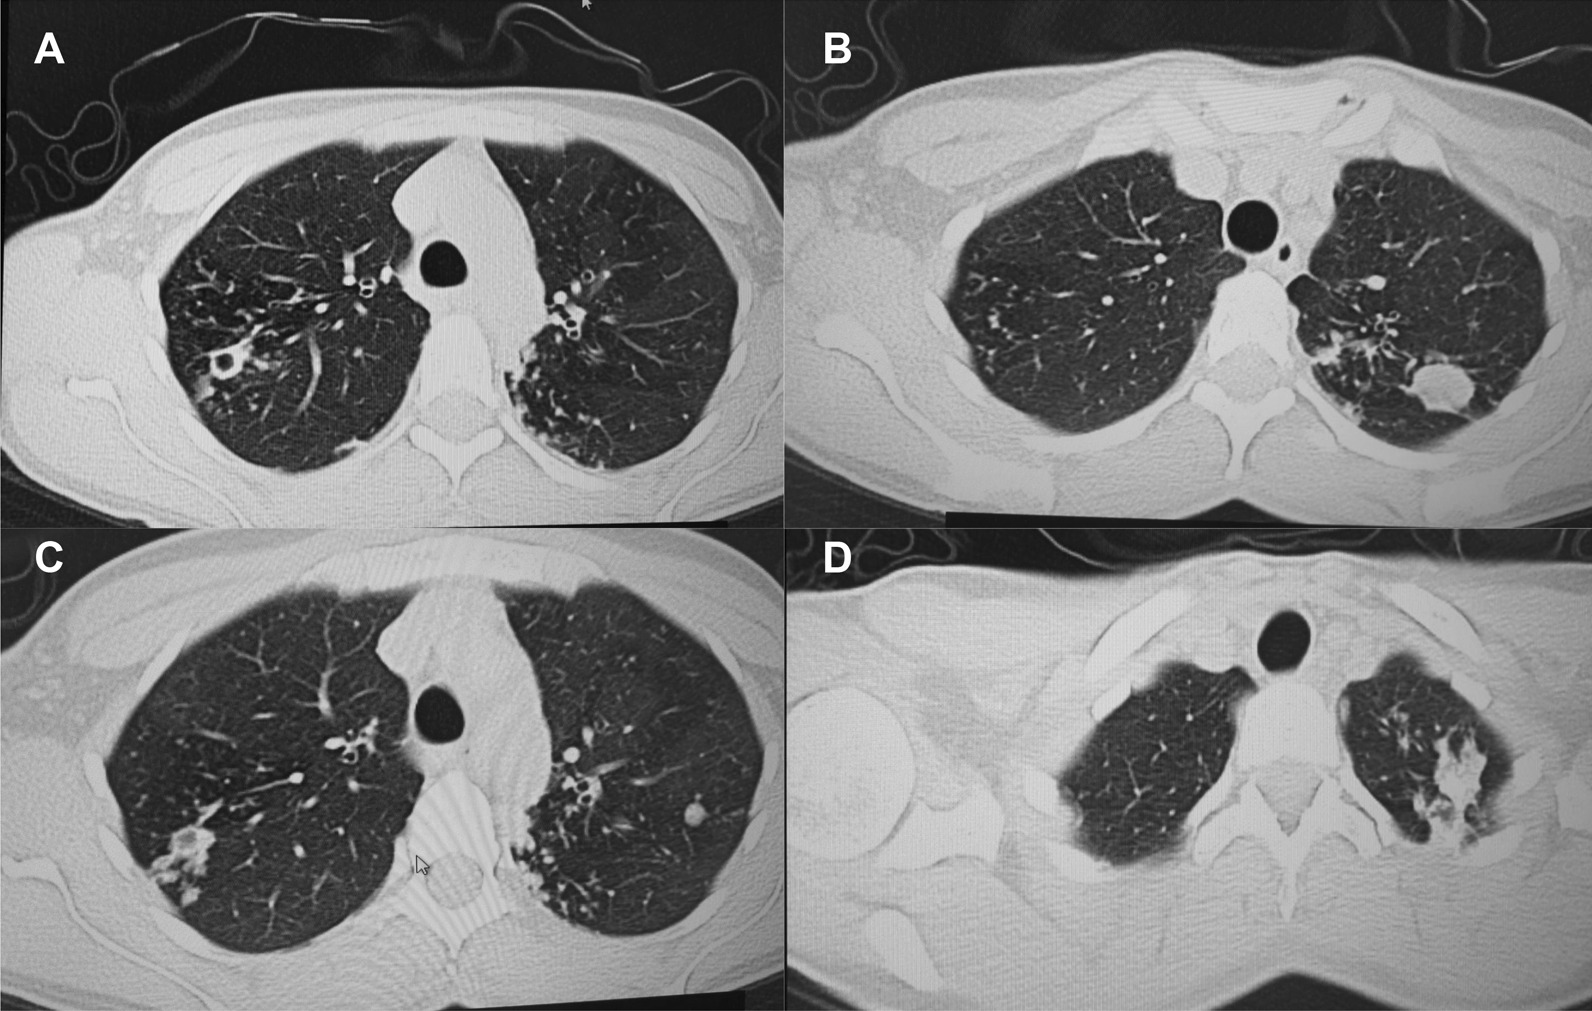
CT scan (January 11, 2021) showing, A a cavity in the upper lobe of the right lung with heterogeneous thick walls. B S1 and S2 segments of the left lung shows a 23 × 18 mm oval shaped calcified inclusions; C, D areas with calcified, compacted nodules 13 × 20 mm in size with additional traction bronchiectasis
